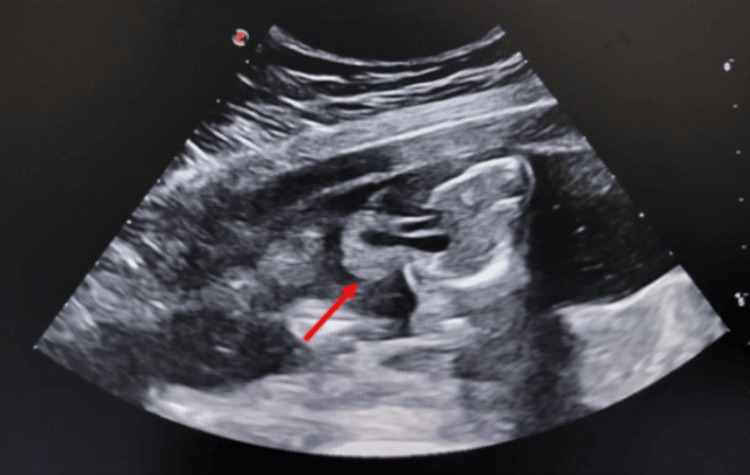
Antenatal ultrasound showing thoracic wall defect with ectopia cordis. B-mode antenatal ultrasound, axial plane at the level of the fetal chest demonstrating a midline anterior thoracic wall defect with partial ectopia cordis (red arrow).
